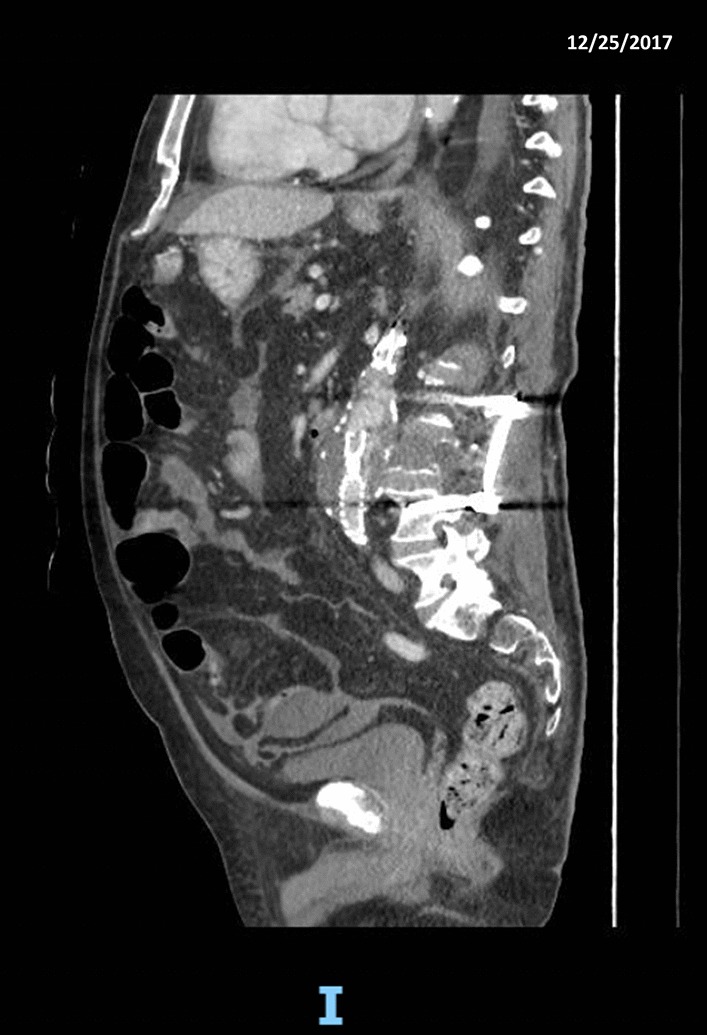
CT imaging showing the contiguity between the aortic sac and the affected vertebra (after the L2–L4 arthrodesis)
